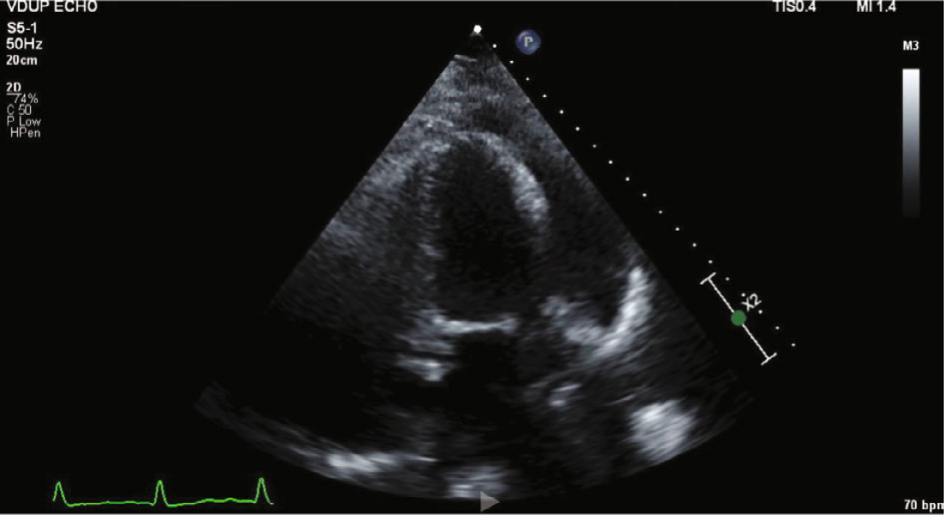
Apical Four-Chamber view on transthoracic echocardiography showing a 1–2-mm pericardial effusion.
